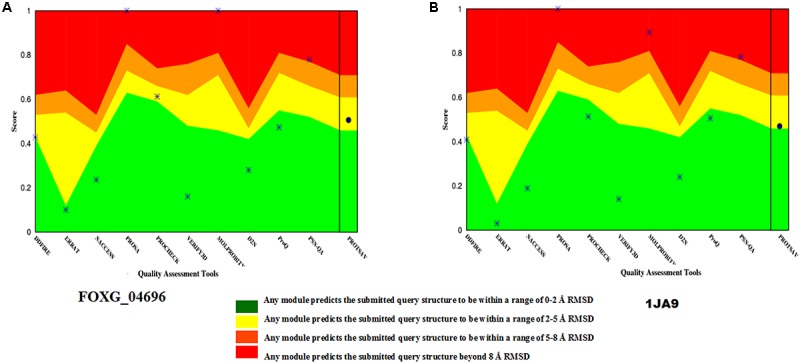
The qualitative assessment of the predicted model FOXG_04696 and its comparative evaluation with the X-Ray resoluted template proteins (1JA9) using the ProtSAV score. (A) The qualitative assessment of the modelled protein (FOXG_04696) based on ProTSAV score. (B) The ProTSAV score for the template protein 1,3,6,8-tetrahydroxynaphthalene reductase (T4HNR) complexed with NADPH and pyroquilon (1JA9). The ProTSAV evaluated the predicted model structures, based on some popular online servers and standalone tools, and furnishes with a single quality score in case of individual protein structure, along with a graphical representation and ranking in case of multiple protein structure assessment. In our results, the ProTSAV score was found close to 1JA9 which predicts the model has reasonable stability and accuracy in terms of qualitative and quantitative parameters.
